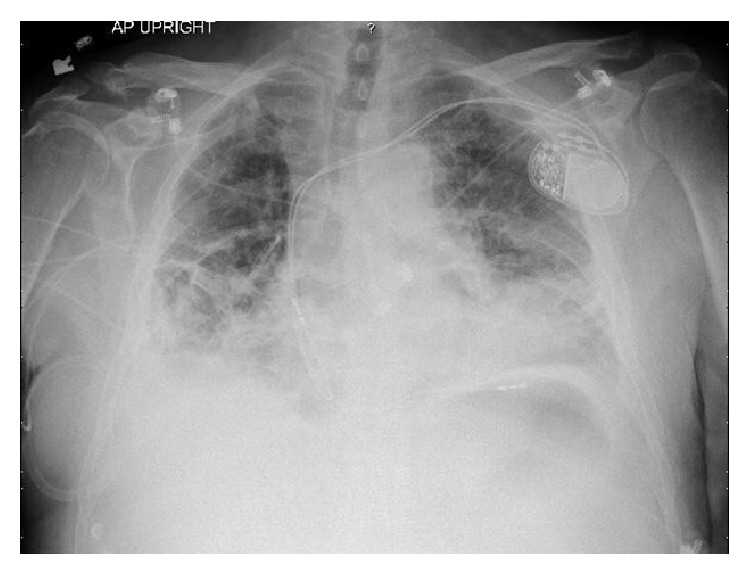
Chest X-ray on presentation showing bilateral lower lobe consolidations.
